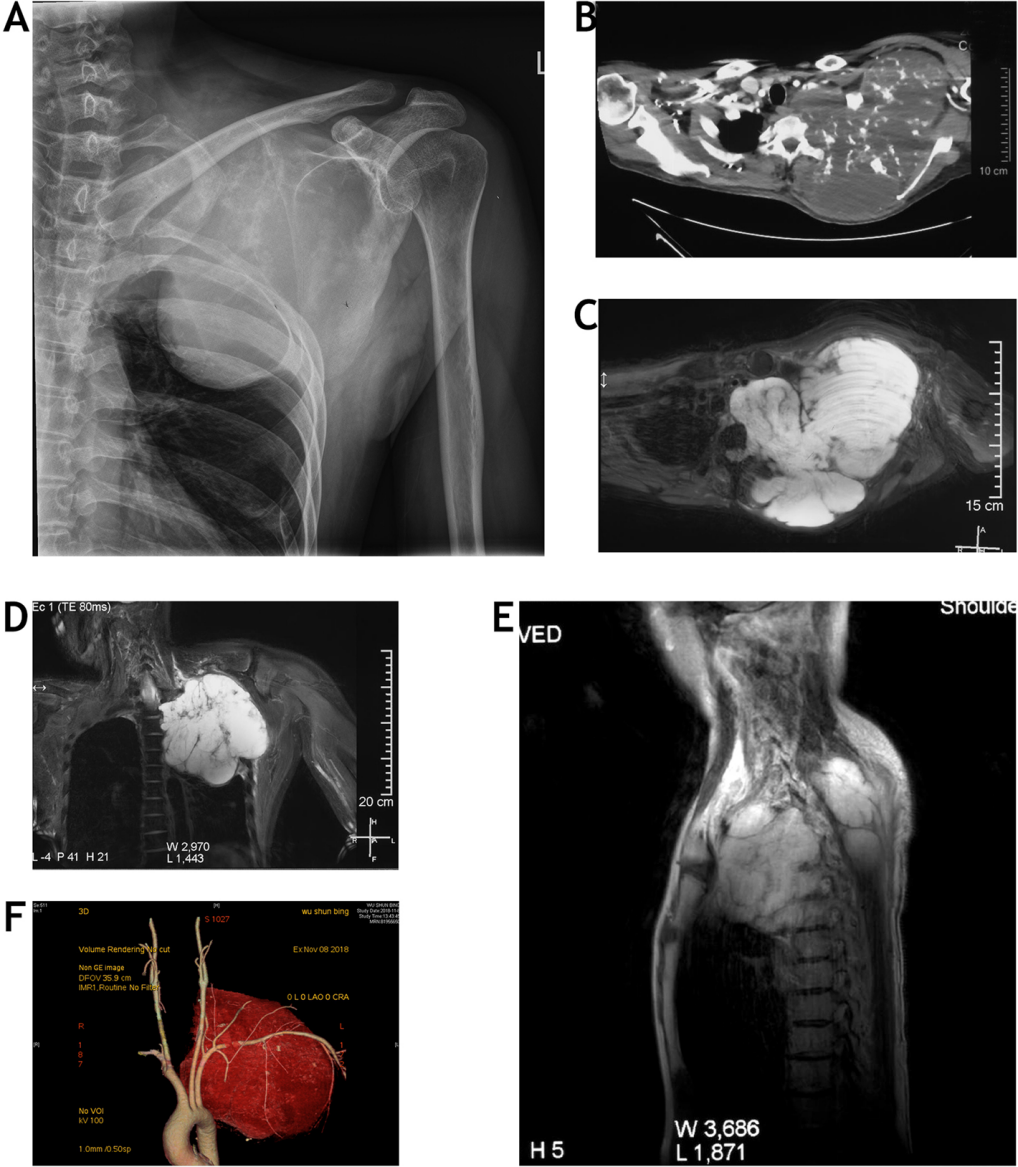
The preoperative plain radiograph (a), enhanced computed tomography (b), T2-weighted axial view (c), coronal view (d), and sagittal view (e) images show tumor involvement of T1-T5 and left thoracic cavity. The left subclavian artery is compressed by tumor on three-dimensional image reconstruction (f)
